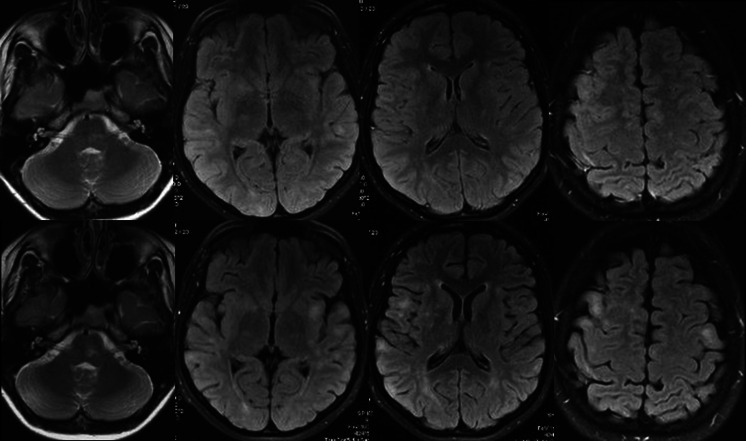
Axial T2- and T2-FLAIR images, two MRI examinations in case 2. *Bottom row* shows progression of lesions seen after 2 weeks
